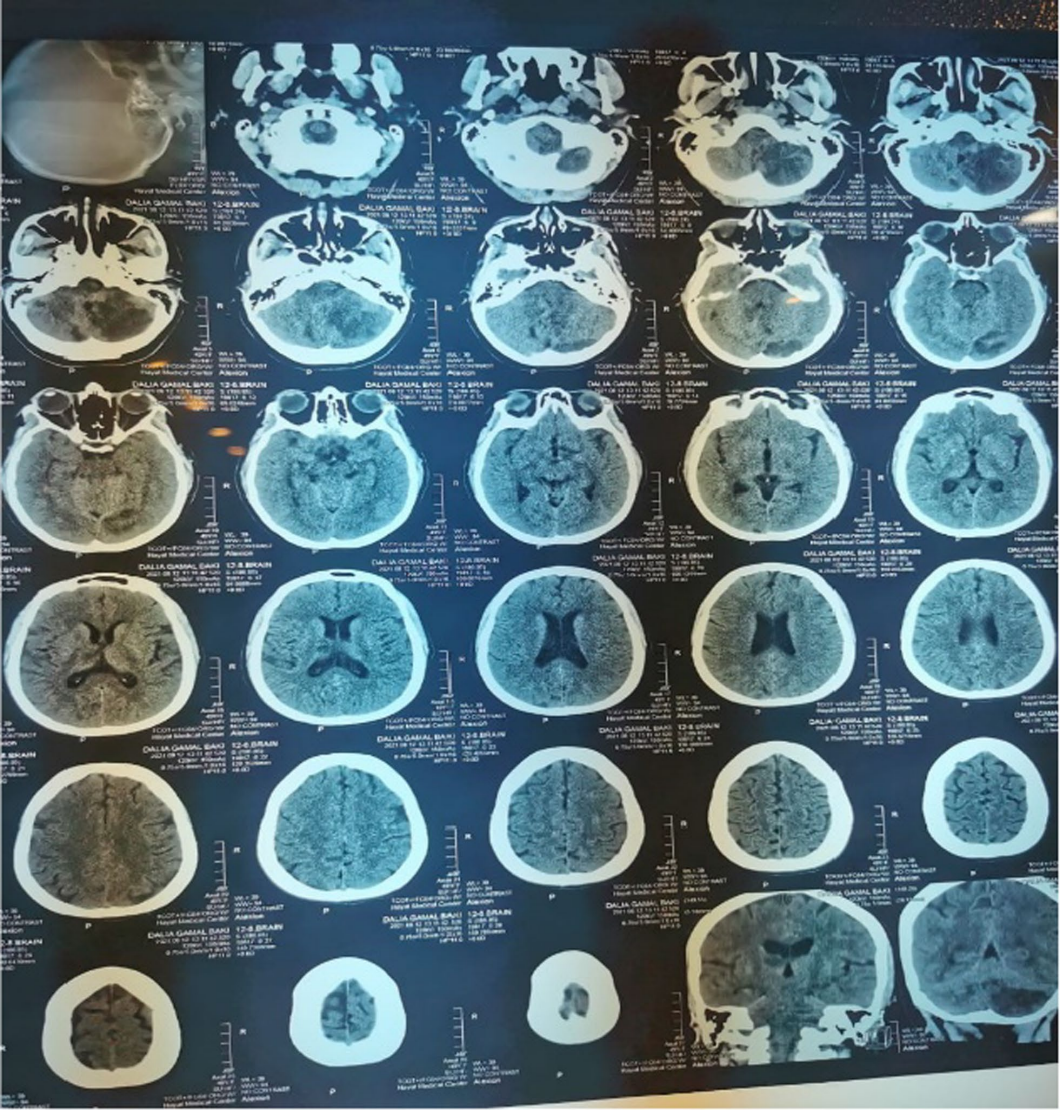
CT of the brain was done and showed left cerebellar, left occipital as well as right thalamic recent infarctions
